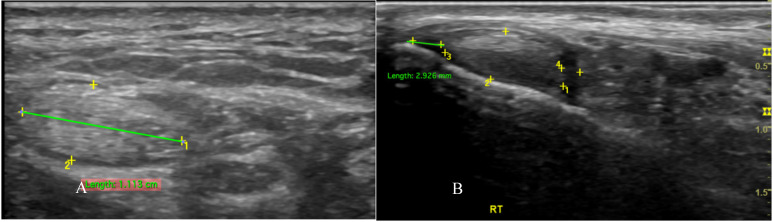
Transverse sonographic of posterior tibialis tendon with tenosynovitis showed thickening of the tendon fiber (A) with fluid in the tendon sheath (B).
